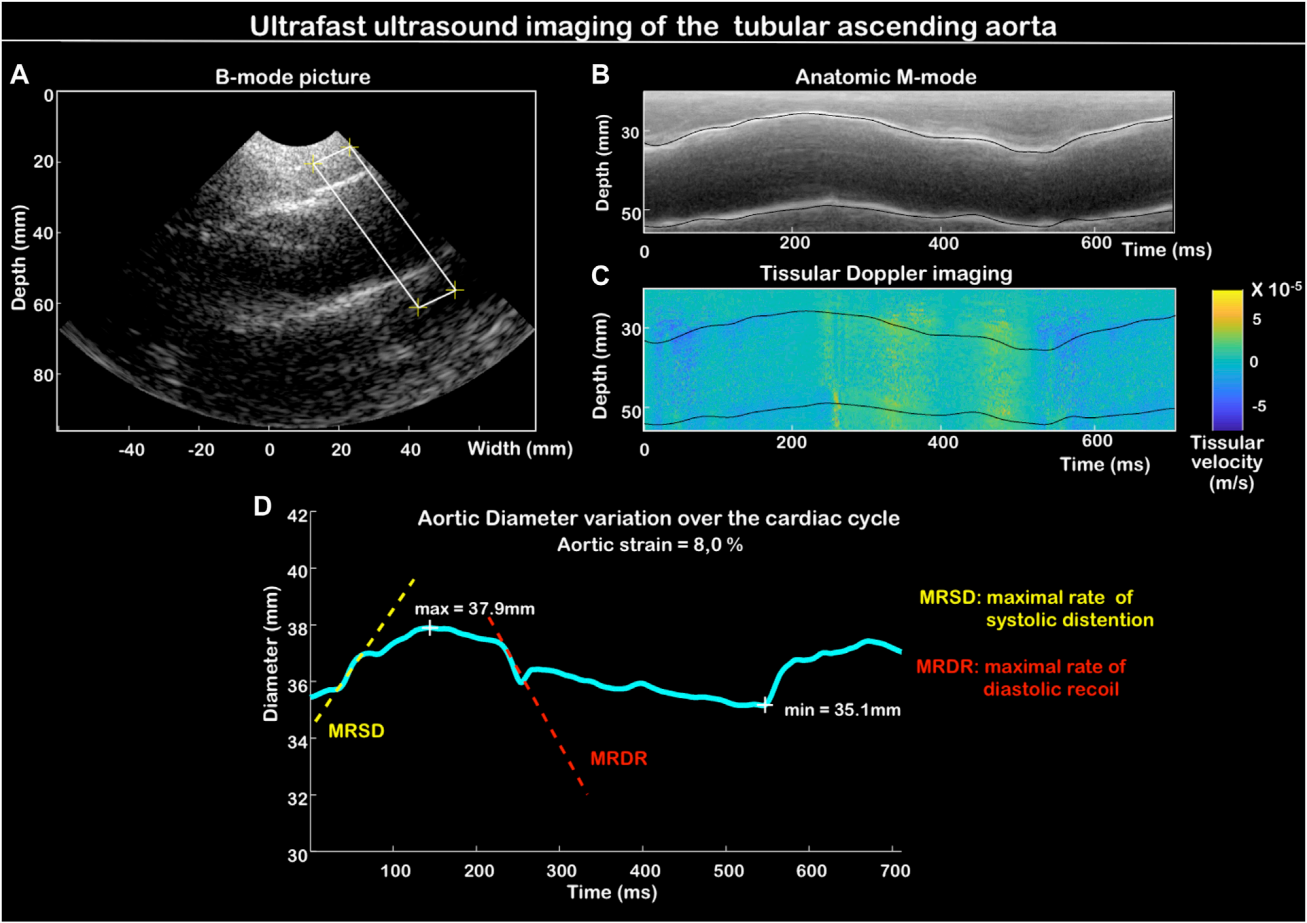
Aortic distensibility measurement method: delimitation of the area of interest of the aorta on the B-mode image. Here at the level of the sinus of Valsalva (A). Automated wall delineation from the anatomic M-mode (TM image) of the delineated segment (B). Collecting tissue velocity values (Tissue Doppler imaging) for each aortic wall over time (time) (C). Determination of the aortic diameter variation curve over time (D).
